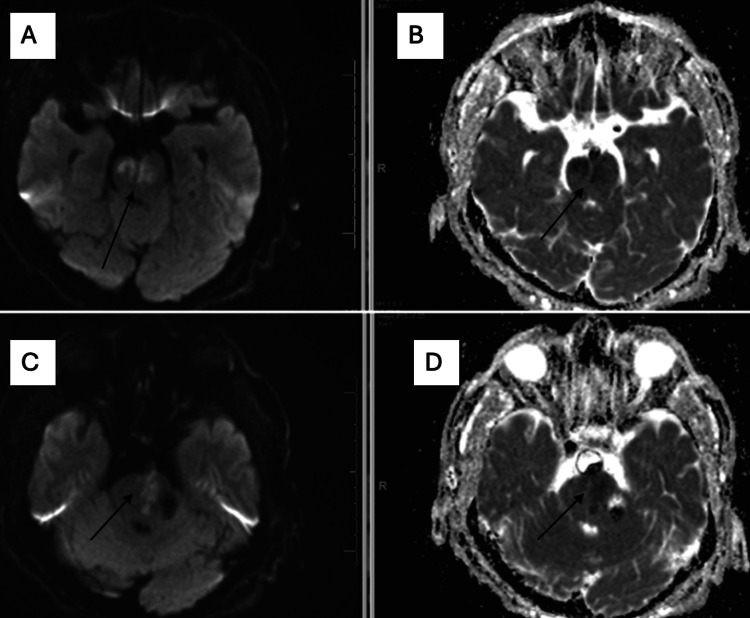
A and C: MRI of the brain without contrast demonstrates large areas of restricted diffusion within the pons, more prominent on the left side. B and C: Corresponding apparent diffusion coefficient (ADC) images show hypointensity in the same regions, consistent with acute infarction.
